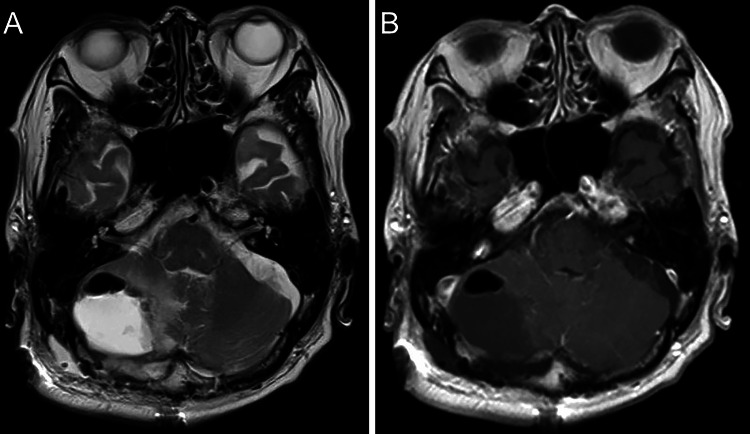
Postoperative MRI (A) Postoperative axial T2- and (B) T1-weighted MRI with (D) gadolinium enhancement reveals no residual tumor in the right posterior fossa. Gross-total removal is achieved. MRI: magnetic resonance imaging
